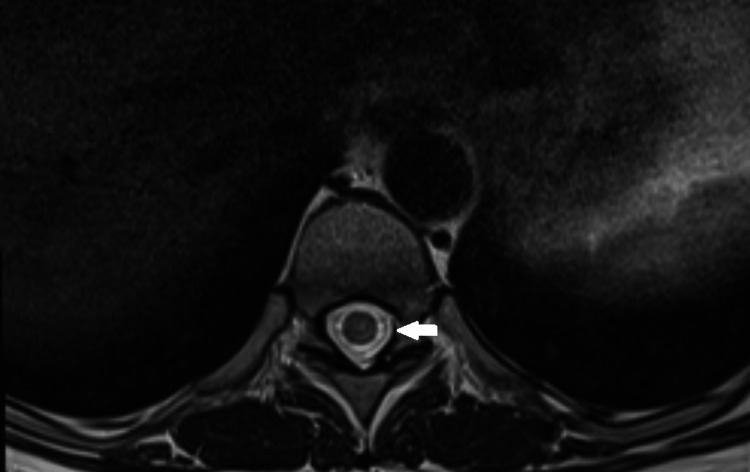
Axial MRI Showing the “Bagel Sign”. Axial T2-weighted MRI of the spinal cord demonstrating the characteristic “bagel sign” (arrow), consisting of a central T3 hypointense core surrounded by a hyperintense rim.
